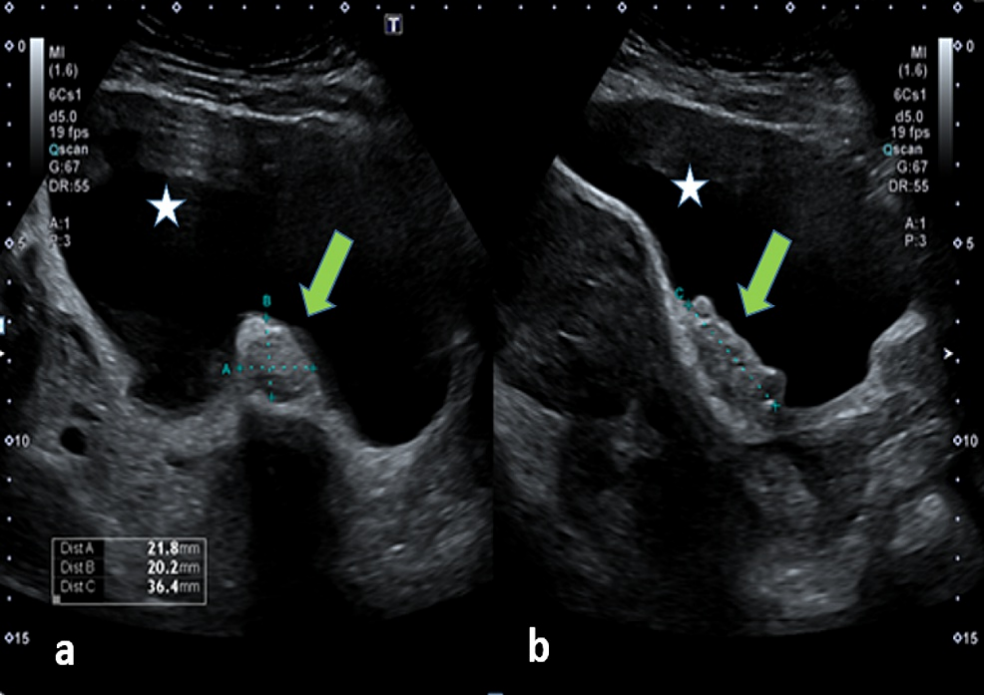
Ultrasound image (a) Transverse and (b) longitudinal image: a well-defined lobulated hypoechoic lesion (green arrow) along the base of the urinary bladder (star sign)
